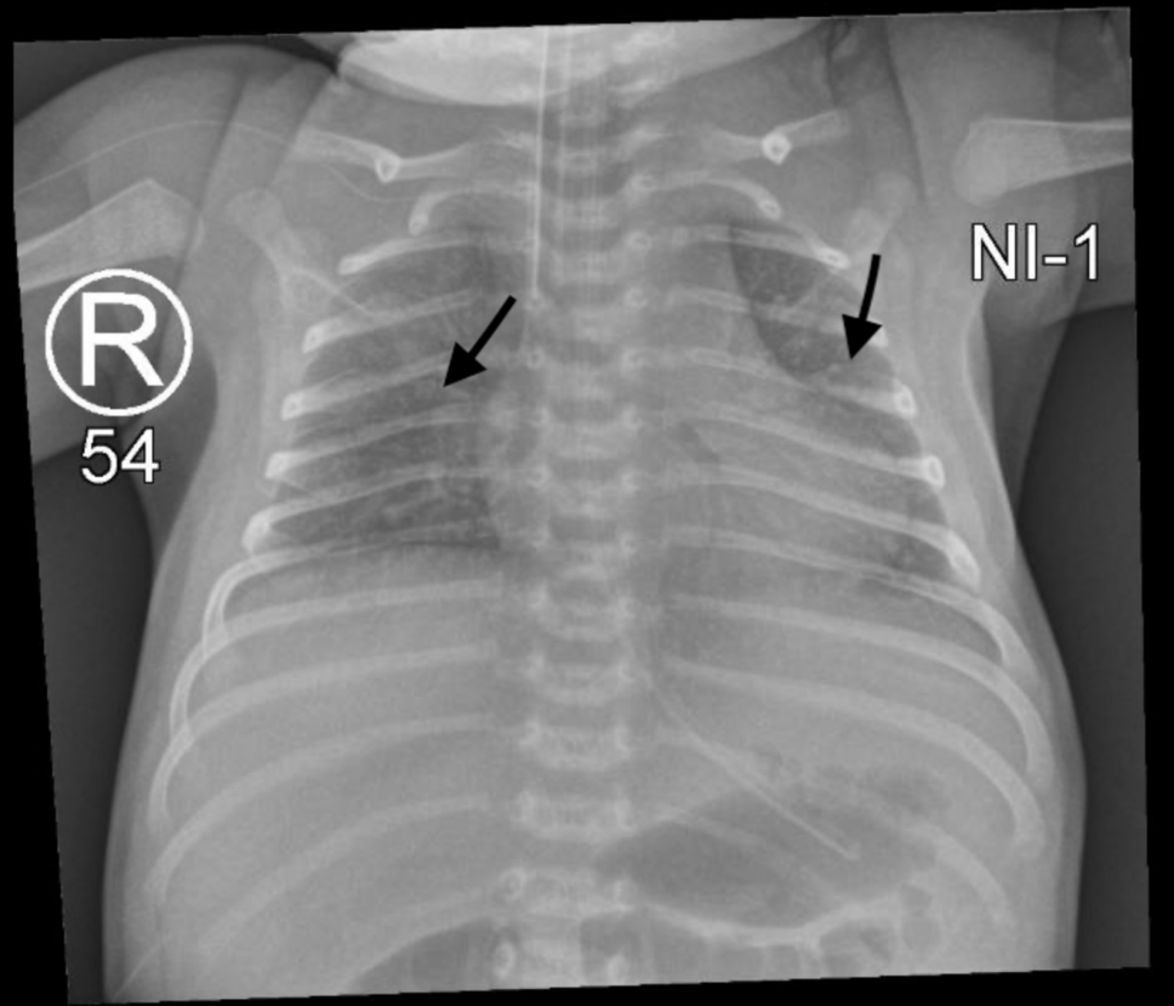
X-ray showing multiple infiltrates
